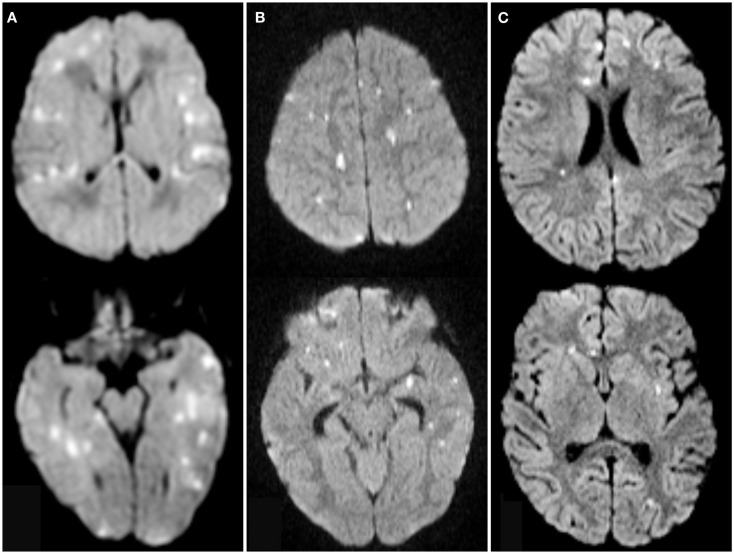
Axial diffusion weighted images from one neonate (A) and two children (B,C) with multifocal punctate ischemic foci without large or medium vessel stroke demonstrating diffusion restriction in multiple ASPECTS territories. Scores were 15 (A), 21 (B), and 11 (C).
